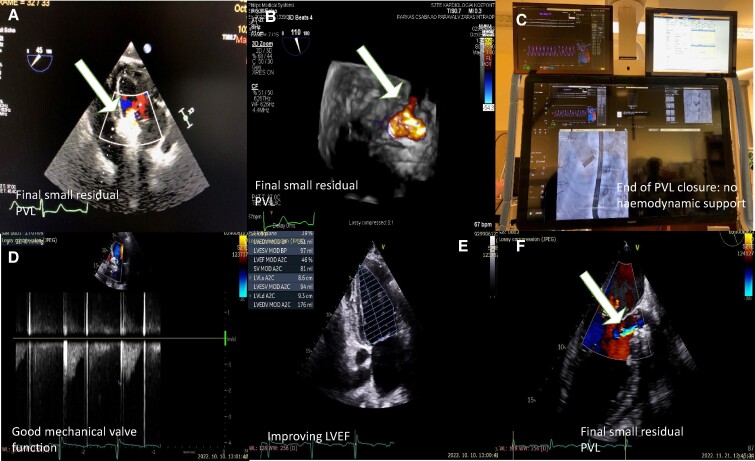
(*A*) Final intraoperative transoesophageal echocardiography demonstrates a good closure result. A white arrow points at the site of plug deployment. (*B*) The final intraoperative three-dimensional transoesophageal echocardiography image demonstrates a good closure result. A white arrow points at the residual small leak. (*C*) Procedure finish, no haemodynamic support. (*D*) Discharge transthoracic echocardiography shows good mechanical valve function. (*E*) Discharge transthoracic echocardiography shows early recovery of left ventricular function. (*F*) Discharge transthoracic echocardiography shows a slight paravalvular regurgitation. A white arrow points at the small residual leak.
